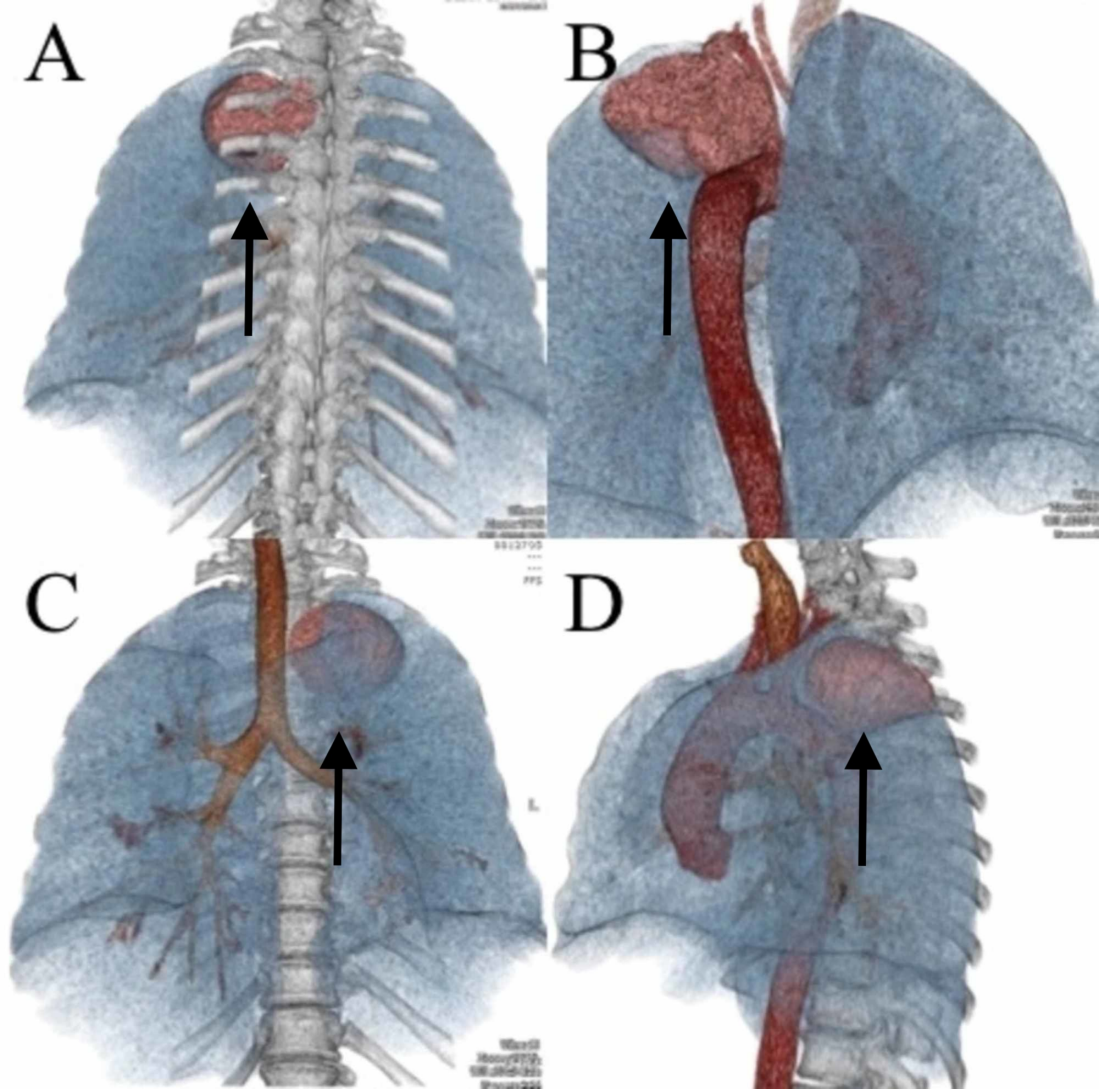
Preoperative 3D CT thorax reconstruction Posterior view (A), posterolateral view with vertebral column removed (B), anterior view (C), and lateral view (D) 3D, three-dimensional; CT, computed tomography
